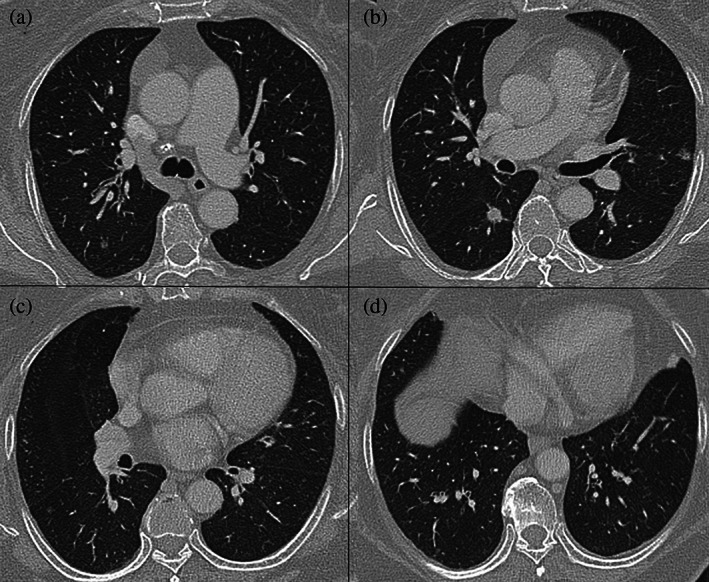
Multiple lung nodules on the CT scan
